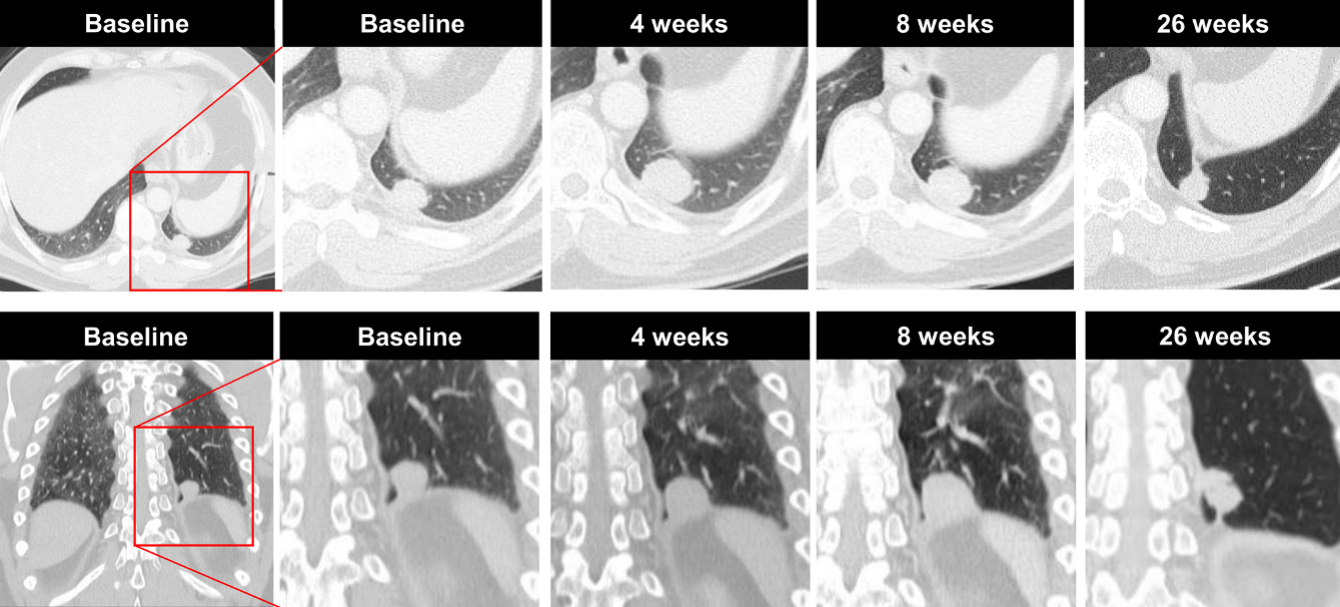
A case of pseudoprogression in a 49-year-old man with NSCLC who was receiving immune checkpoint inhibitor therapy. Coronary CT imaging obtained before therapy demonstrates a 20 mm metastasis in the left lower lobe and 4 weeks after treatment initiation, the tumor size increased from 20 to 29 mm. Therapy was continued, and 4 weeks thereafter, the tumour size was reduced by 4 mm to 25 mm. Treatment was continued and 26 weeks after treatment initiation, the tumor continued to reduce in size, measuring 14 mm. No further metastases were visible at any time point. Upper row: axial view, lower row: cornonal view
